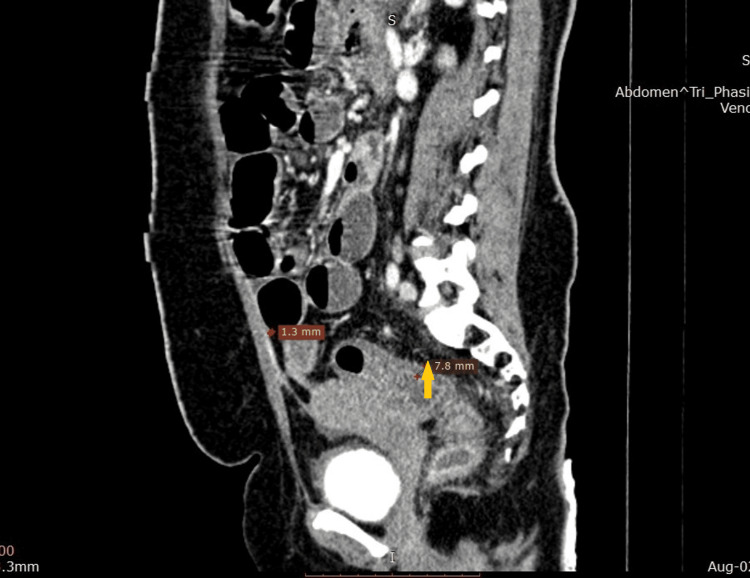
CT of the abdomen showing bowel wall thickening (yellow arrow).
